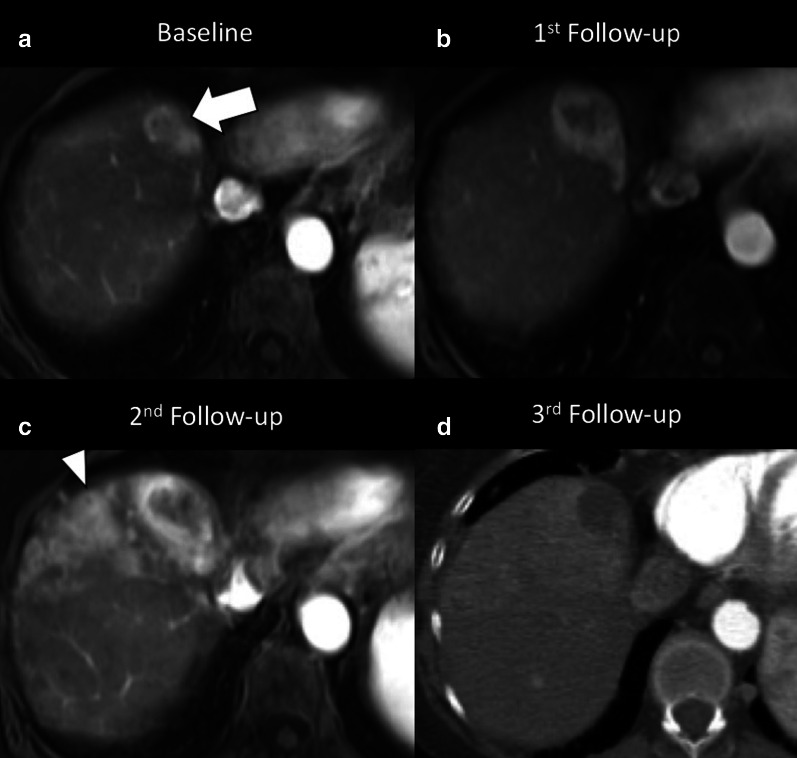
65-year-old woman with viral cirrhosis and hepatocarcinoma previously treated with transarterial embolization with post-treatment persistence of viable tumor (LR-TR viable), then submitted to stereotactic body RT with radiation dose of 40 Gy in 5 fractions. a Contrast-enhanced MRI performed before stereotactic body RT shows the hypervascular HCC (arrow) on arterial phase. b Contrast-enhanced MRI performed two months after treatment showed a size increase of the lesion with reduced enhancement in the central area. c Contrast-enhanced MRI performed four months after stereotactic body RT shows an ill-defined peritumoral enhancement consistent with radiation induced liver disease, that prevents from adequate assessment of tumor margins; the lesion itself shows greater central hypointense area with peripheral enhancement. d Contrast-enhanced CT performed 6 months after stereotactic body RT shows a markedly reduced enhancement of the tumor, as well as reduction of peritumoral enhancement. Therefore, the tumor increase in size in the first follow-up at 2 months was not a true progressive disease and was likely related to RT-related changes. *Case courtesy of Dr. Daniele Marin, Duke University Medical Center, Durham (NC), USA*
